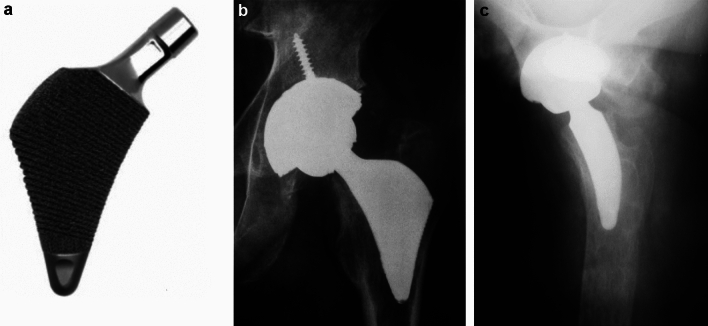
a–c Images of Proxima ultra-short stem. a Photograph of Proxima stem. Note the lateral coronal flare, which creates a symmetrical wedge design in the AP plane. The majority of the stem is coated with a porous coating and covered with hydroxyapatite. b–c Radiographs of the Proxima stem at 11.5 years. Note on AP view b how the lateral flare engages the lateral metaphyseal cortex, and the stem tip does not engage into the femoral diaphysis. Lateral view c again shows no diaphyseal engagement.
